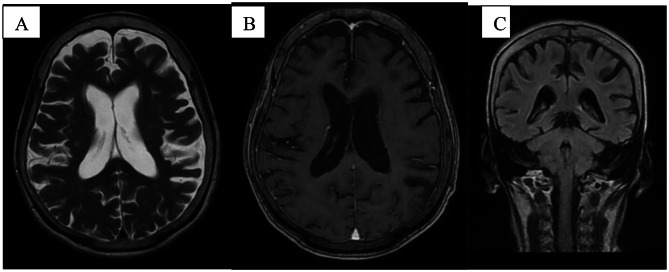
Neuroimaging findings during follow-up in 2months. (A) MRI T2W image showing interim resolution of prior parieto-occipital hyperintensity changes. (B) Post-contrast T1-weighted demonstrating resolution of leptomeningeal enhancement in the left parieto-occipital lobe. (C) FLAIR sequence showing resolution of previous hyperintensities over bilateral parieto-occipital lobes
